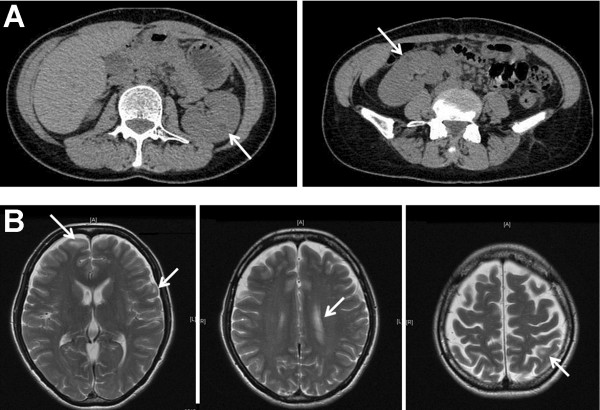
Evidence for kidney and brain involvement on computed tomography and magnetic resonance imaging.  (A) A non-enhanced abdominal computed tomography scan showing bilateral low-density areas with negative Hounsfield unit values inside the kidney, suggesting a renal angiomyolipoma (arrows). (B) Brain magnetic resonance imaging demonstrating multiple small, nodular, T2 high-intensity areas in cortical, subcortical, and subependymal zones, suggesting cortical and subcortical tubers (arrows).
